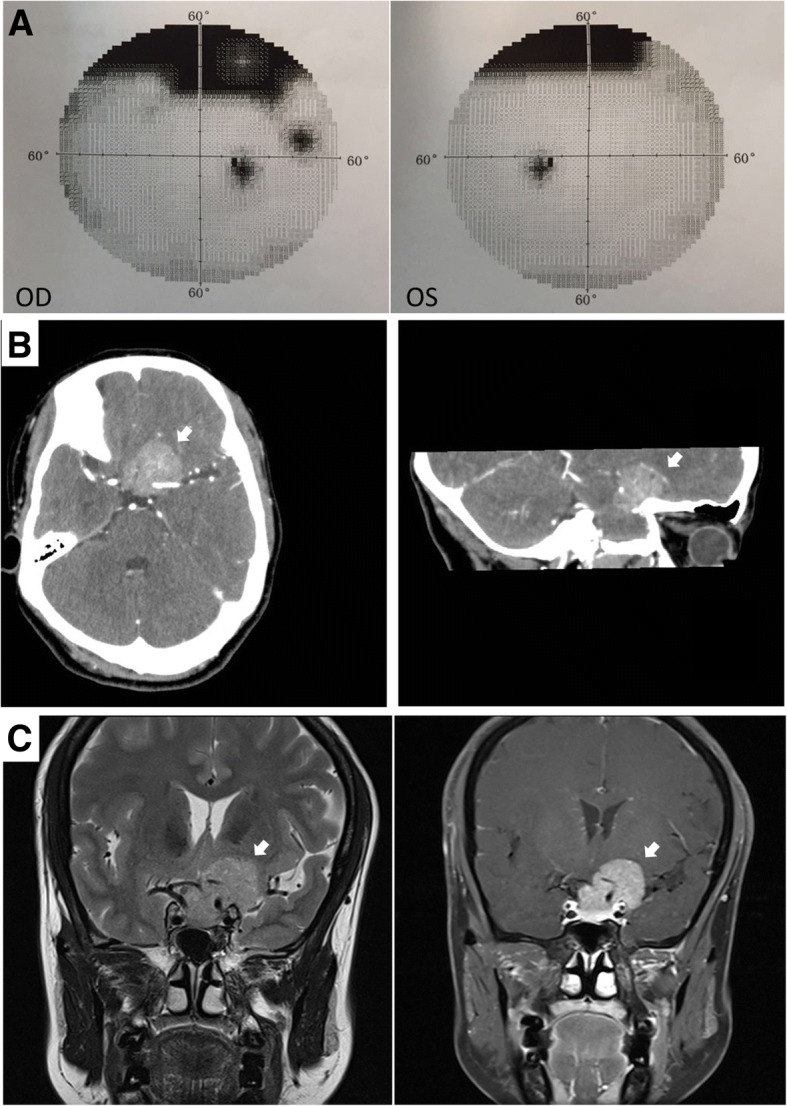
a: Visual field: the superior visual field was narrowed in both eyes, considering caused by drooping upper eyelids. b: Brain CT: showing a mass in sellar-suparsellar-parasellar region (white arrow). c:Brain MRI: showing an irregular soft tissue mass with uniform T1 and long T2 signal(white arrow), which was lobulated, with clear boundaries and compression on the left temporal lobe of the brain
